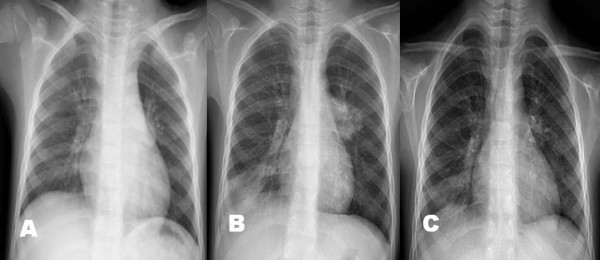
The chest radiographs of a patients who had progressive pneumonia during hospitalization (male, 6-year old); on admission (Figure 3-A), before methyprednisolone (MP) treatment (2nd hospital day, Figure 3-B), and 1 day after MP treatment (Figure 3-C).
